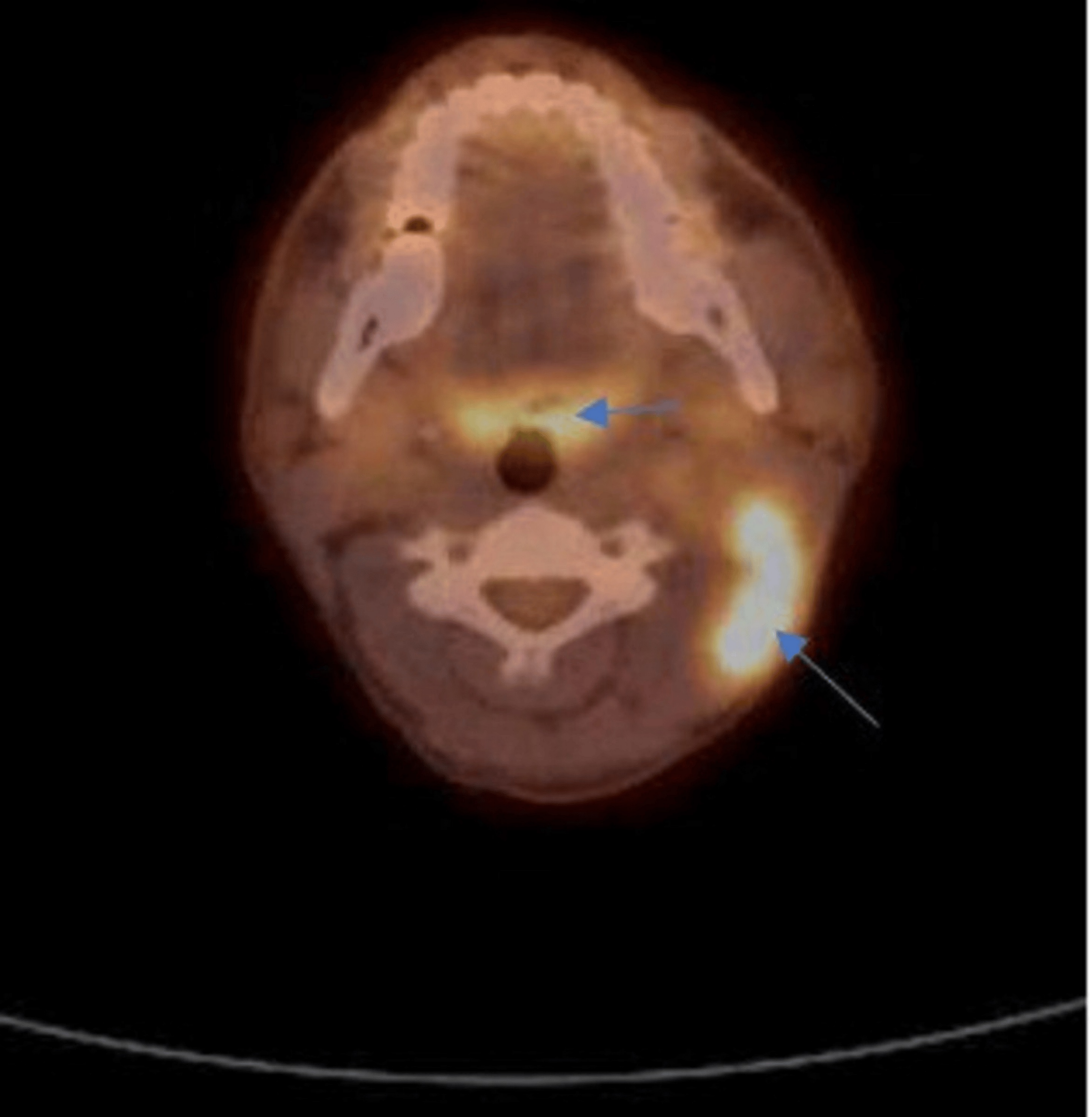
FDG PET-CT of the neck demonstrating hypermetabolic activity in the right upper cervical lymph node and the pre-epiglottic area (blue arrows). FDG PET-CT: fluorodeoxyglucose positron emission tomography-computed tomography
